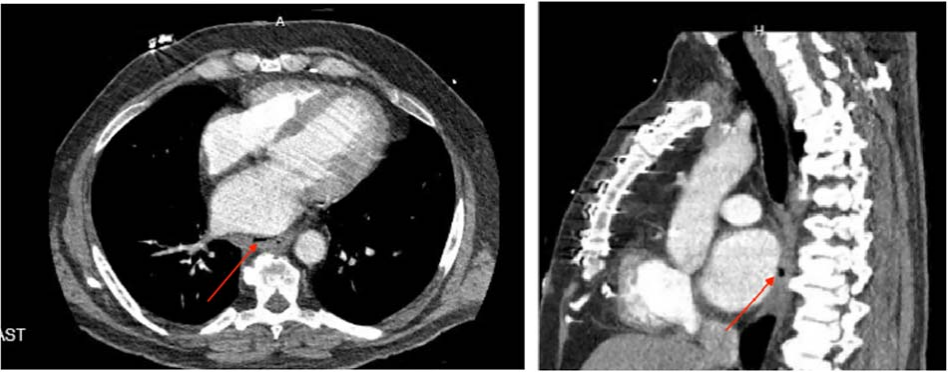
(a) Axial CT image of pericardioesophageal fistula of Case 4 (arrow indicates area of fistulization); (b) sagittal CT image of pericardioesophageal fistula of Case 4 (arrow indicates area of fistulization).
